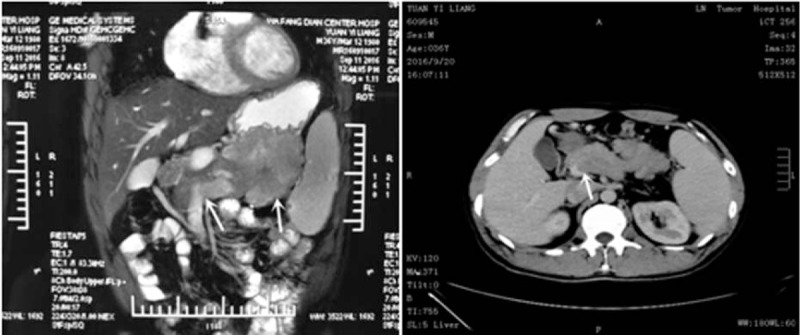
Preoperative MRI and CT scans of our patient indicated a tumor (9.6 cm × 7.6 cm) in the pancreatic tail closely related to the stomach and spleen, and portal vein thrombosis. CT = computed tomography, MRI = magnetic resonance imaging.
